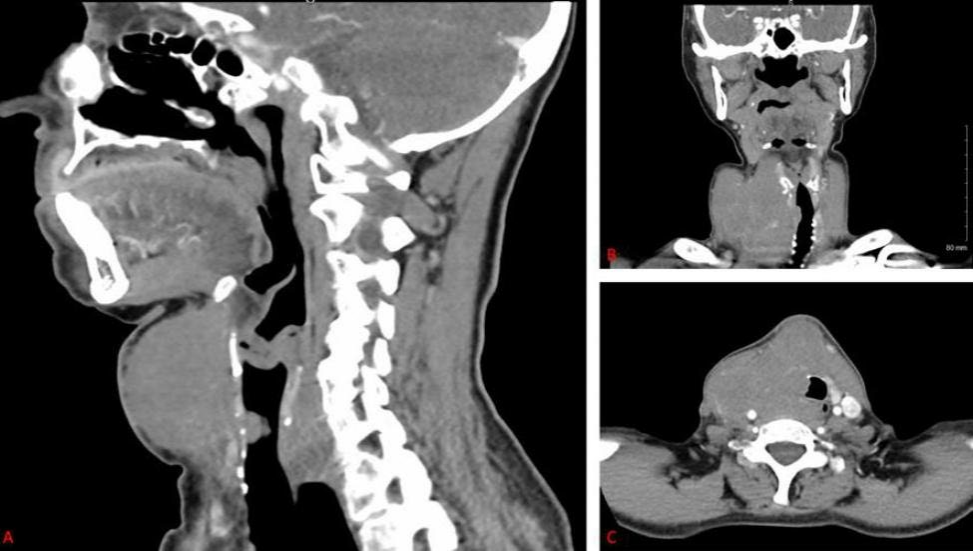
Sagittal (A), coronal (B), and axial (C) images from a computed tomography of the neck revealing an 8-centimeter mass within the thyroid gland with destruction of the thyroid cartilage and evidence of narrowing of the cervical trachea
